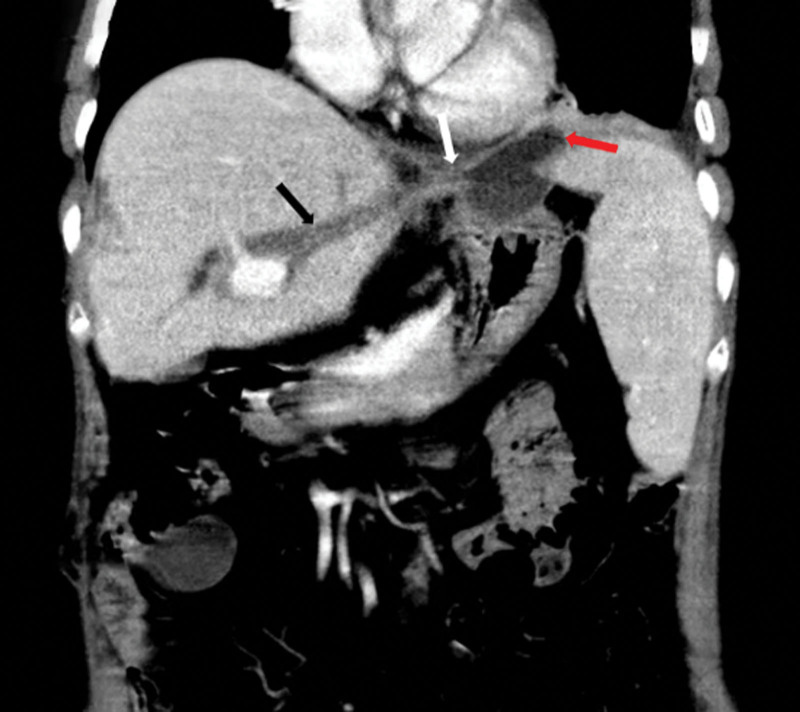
CT image scans revealed intrahepatic bile duct calculi, and biliary tract dilation (Black arrow); dilated bile duct is connected to the subphrenic abscess (White arrow); and the subphrenic abscess contained gas (red arrow). CT = computed tomography.
